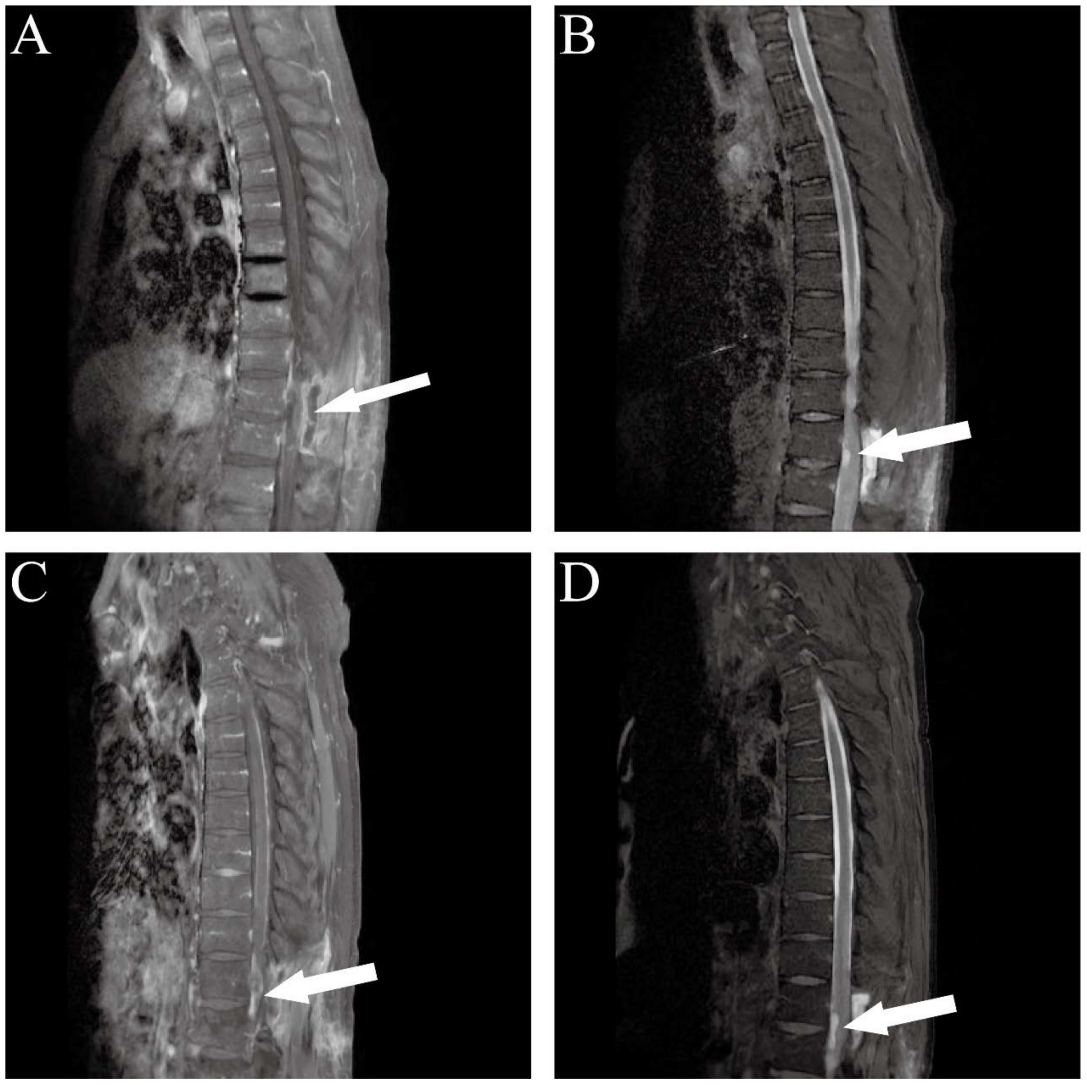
Dynamic postoperative MRI follow-up of the thoracic spine. (A, B) Early postoperative images. (A) Sagittal contrast-enhanced T1-weighted imaging (T1WI+C) showing postoperative epidural fluid collection and soft tissue swelling at the T11–T12 level. (B) Sagittal T2-weighted fat-suppressed imaging (T2-FS) demonstrating hyperintense fluid collection at the surgical site with surrounding inflammatory high signal. (C, D) Follow-up imaging approximately 1.5 months after surgery. (C) Sagittal T1WI+C reveals an enhancing intradural nodule at T12 level, indicative of tumor recurrence. (D) Sagittal T2WI demonstrates the nodule with mildly prolonged T2 signal and enlargement of the epidural linear high-signal area.
